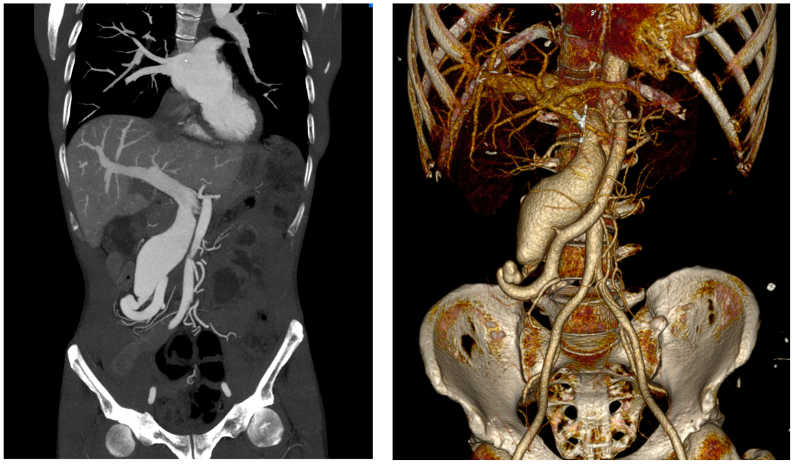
Computed tomography angiography findings. (Left) Two-dimensional coronal image showing dilatation of vascular lesion enlargement to the hepatic vasculature. (Right) Three-dimensional image showing the superior mesenteric artery (SMA) and dilated superior mesenteric vein (SMV) connected by a fistula.
